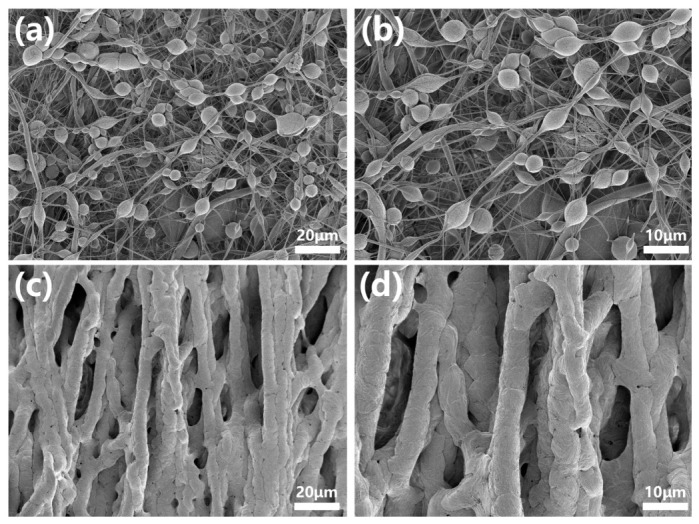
Multi-layered fibrous structure where upper fibers drape over lower fibers, creating a complex web of interconnected pores with significant overhangs. (a,b) SEM images of surface morphology for the conventional fibres with different magnifications. (c,d) SEM images of surface morphology for the 3D porous structure with different magnifications caused by the simultaneous ultrasonic and magnetic field.
